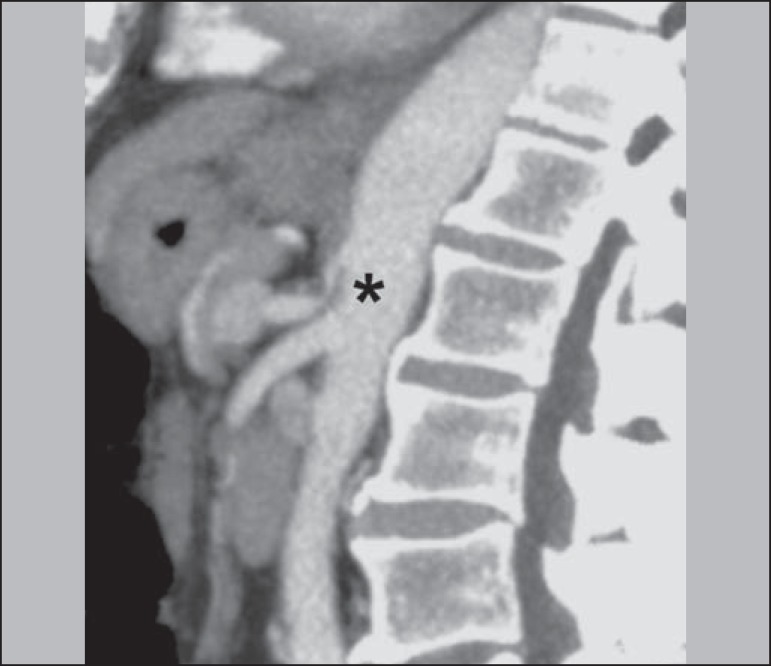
Median arcuate ligament syndrome. Sagittal CT showing a "hooked" aspect
